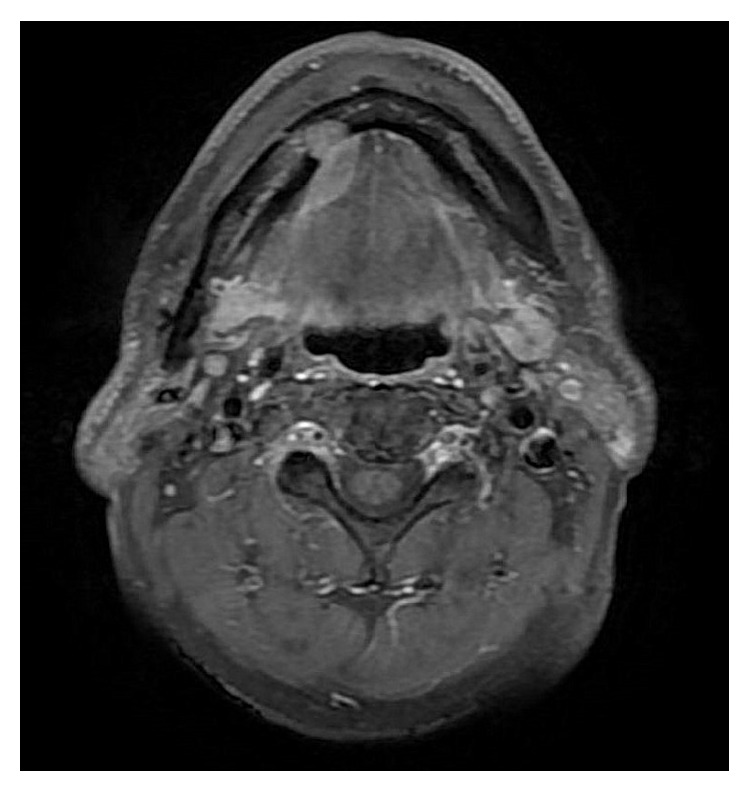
Magnetic resonance imaging (MRI) shows that both bone cavities are filled with soft tissue. The lesion in the premolar region is caulescent from the sublingual gland with identical signal intensity to the right sublingual gland, showing a contrast effect compared to the left sublingual gland. The lesion at the angle of the mandible seems to be part of the submandibular gland.
